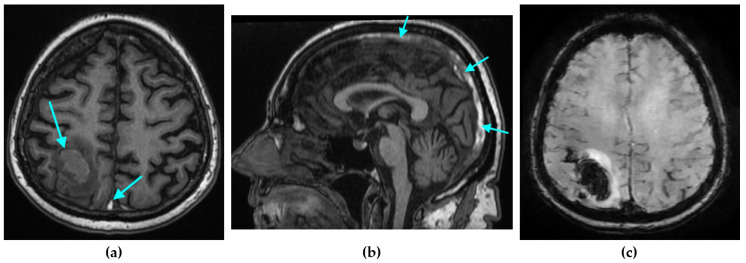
Acute-phase non-contrast brain MRI demonstrates a hemorrhagic venous infarction in the right parietal lobe and associated thrombosis of the superior sagittal sinus (arrows) with (a) axial T1-weighted image, (b) sagittal T1-weighted 3D BRAVO sequence highlighting the superior sagittal sinus and (c) axial 3D SWAN sequence demonstrating susceptibility changes associated with hemorrhage.
